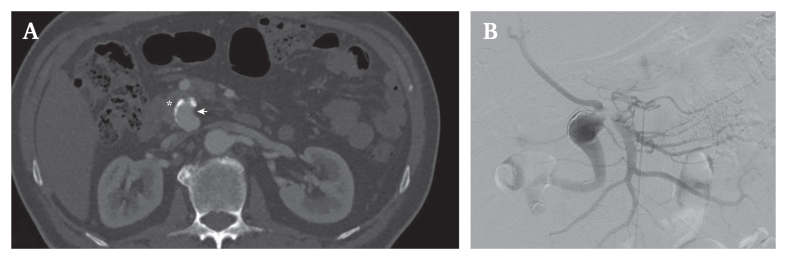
(A) Computed tomography angiography (CTA). The axial pre-surgical CTA view showed an inferior pancreaticoduodenal artery (iPDAA) (arrow) in close proximity to the pancreatic head (∗). (B) Digital subtraction angiography (DSA). The DSA confirmed a complete chronic occlusion of the coeliac artery (CA), which appeared independent of respiratory movements (not shown). The endovascular revascularisation attempt failed. The DSA further confirmed the iPDAA with a variant arterial blood supply of the liver. The right hepatic artery (RHA) arises directly proximal to the iPDAA from the superior mesenteric artery (SMA).
